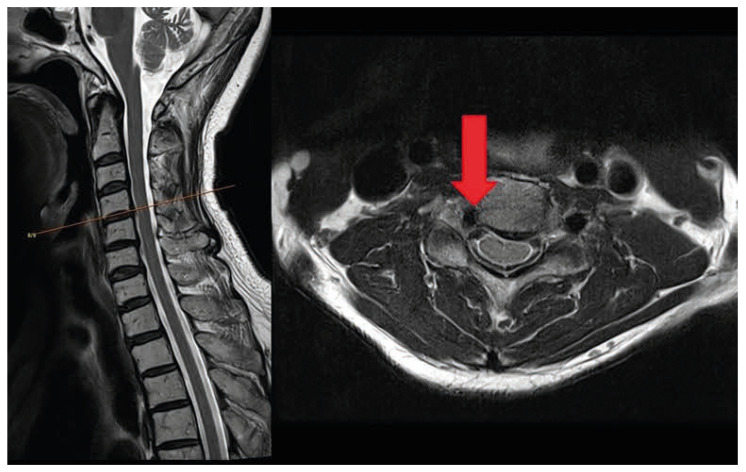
Magnetic resonance imaging of the cervical spine showing a vascular structure occupying the right C4-5 neural foramen (Red arrow).
